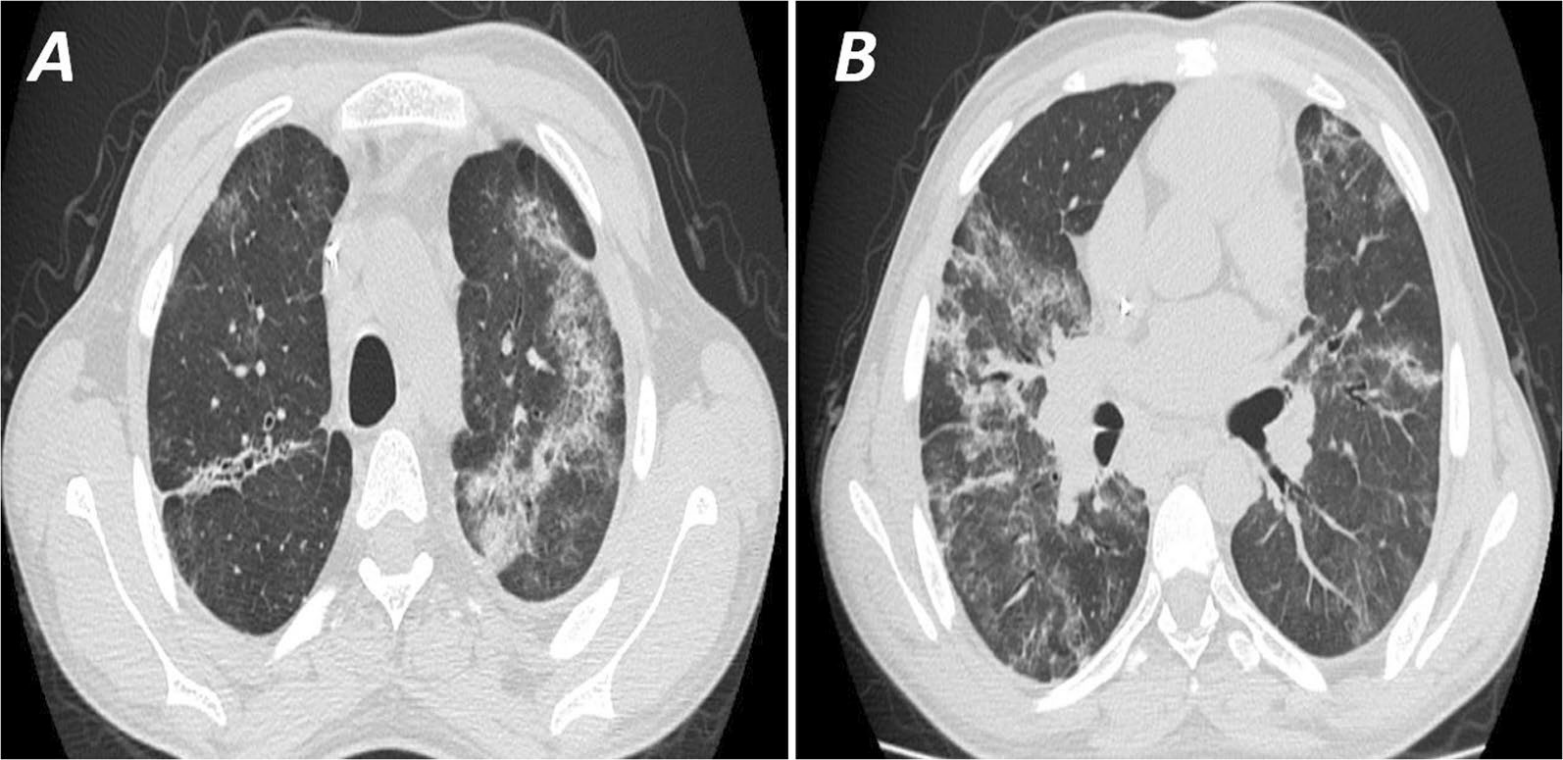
A and B High-resolution CT chest (axial cuts) for a 25-year-old male patient with common variable immune deficiency presented with fever, cough, and dyspnea. CT was interpreted as typical (CO-RADS 5) by all readers. RT-PCR for COVID-19 was positive. The patient improved and was discharged in two weeks
